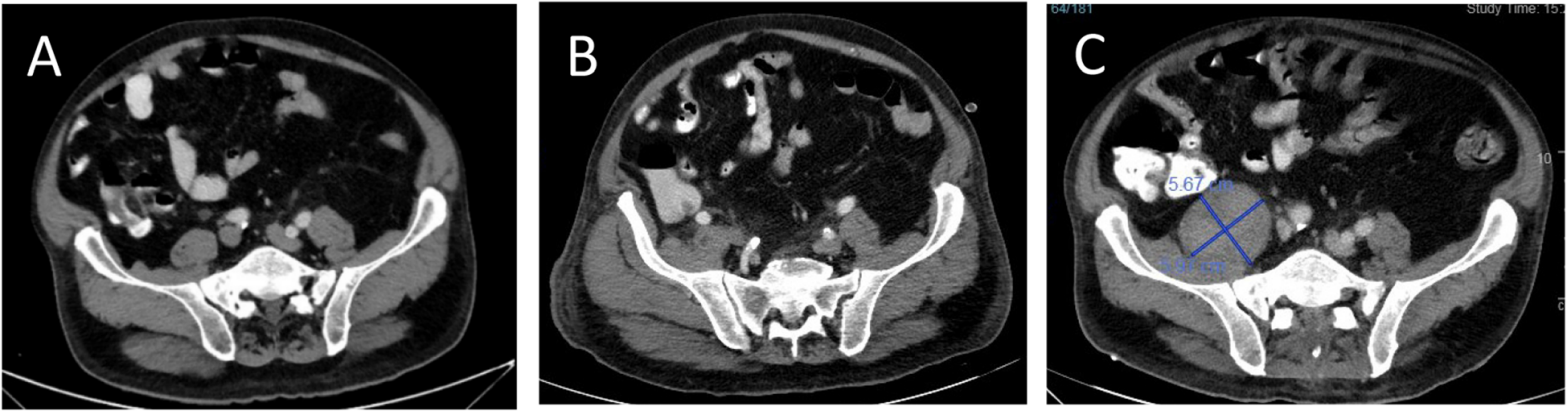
Psoas abscess development on consecutive double contrast abdominal computed tomography scans. Scans were done on days 3 (a) 7 (b) and 26 (c) post- endoscopic retrograde cholangiopancreatography ERCP
